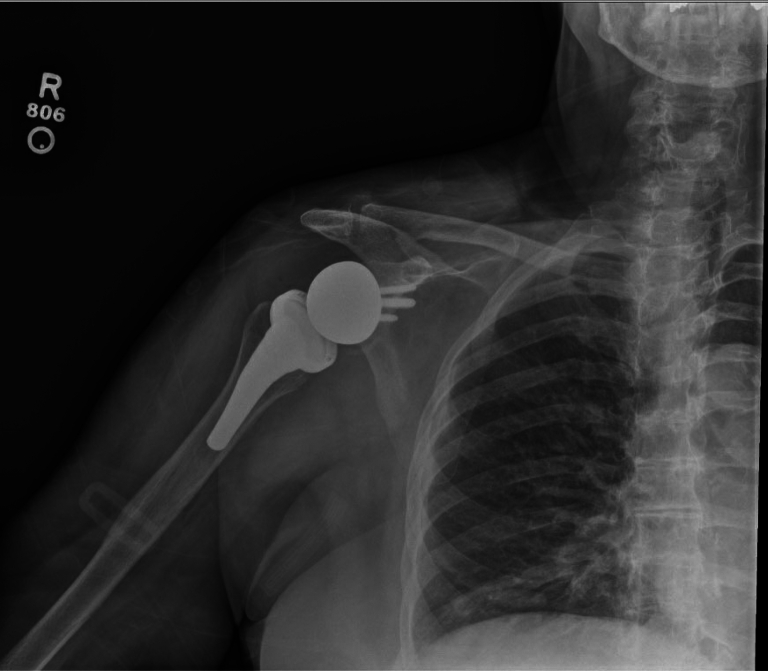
Immediate postoperative radiograph depicting interval postsurgical changes of right RSA. The hardware appeared intact without peripheral lucency. *RSA*, reverse shoulder arthroplasty.
